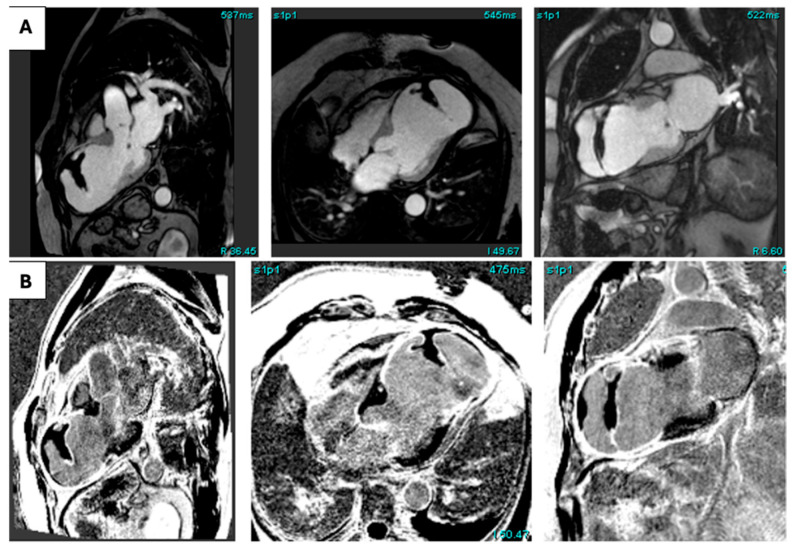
Preoperative cardiac magnetic resonance imaging (CMR). (A) Cine images demonstrating a giant post-infarction left ventricular aneurysm involving the apical and anterior segments, associated with severe left ventricular dilatation and adverse remodelling. (B) Late gadolinium enhancement sequences showing extensive transmural scar formation of the aneurysmal wall and partially organized mural thrombus. A discontinuity within the aneurysmal wall with a thin overlying tissue layer is visible, raising suspicion for a chronic contained free-wall rupture.
